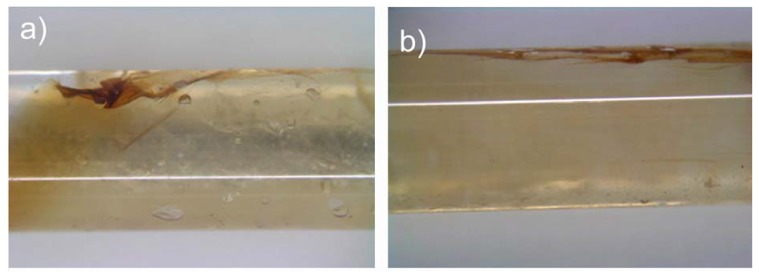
Photographs of the hybrid actuator assembled from films and swollen Cabloc CT hydrogels in a 2 mm diameter glass tube. There are two common defects: (a) the film adheres well to the inner surface but some parts of the film overlap. (b) At lower loading of hydrogel, there are bubbles between the wall and the film. Both structures will result in wrong reconstructions of the inner surface. White lines on the tubes are reflection on the glass from the lamp used to illuminate the samples.
